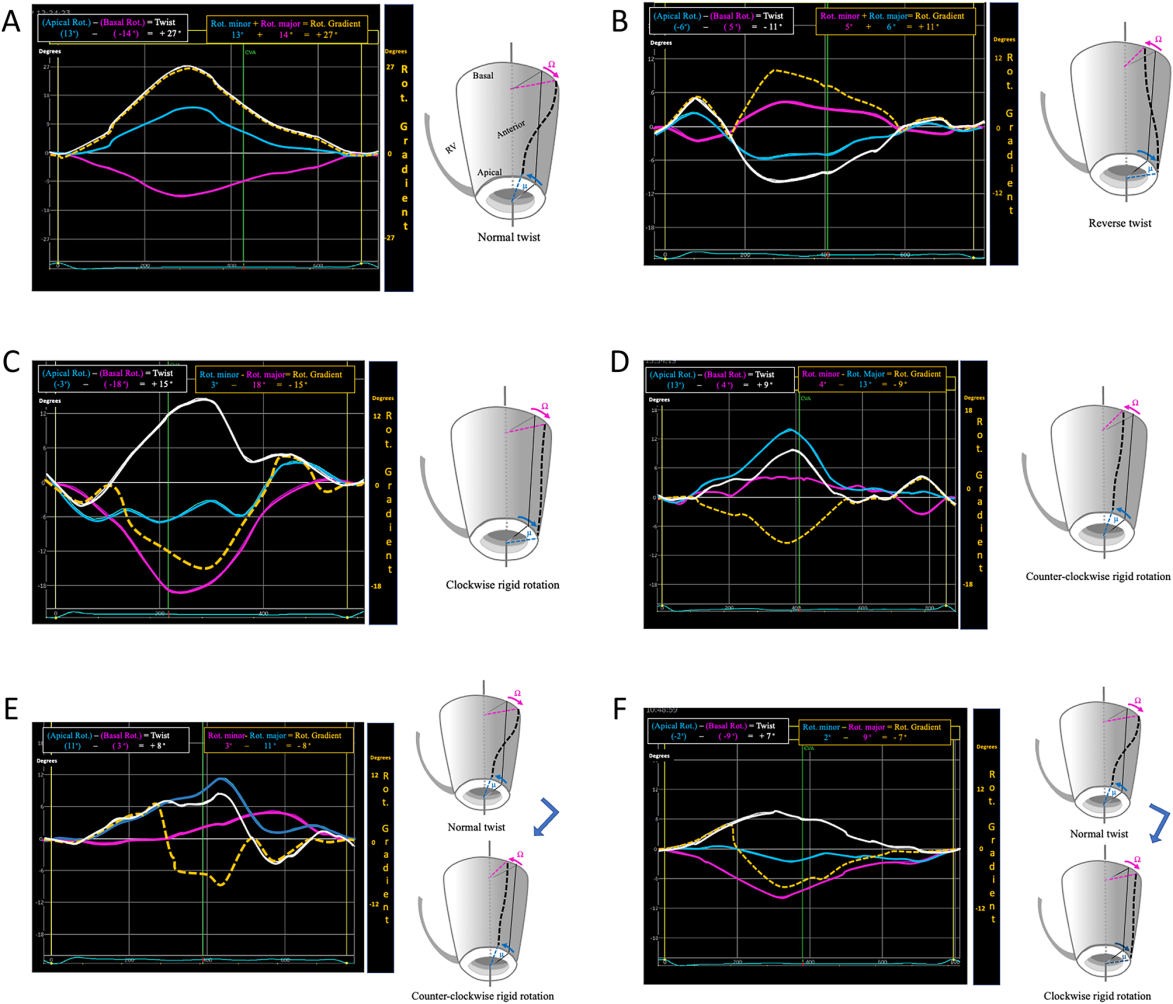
Examples of different rotation models obtained in our study population. Representation according to coding system. With the currently used coding system the curve of rotational gradient is represented as a continuous white line when visualized through an echocardiography device. With the coding system we propose, the curve of rotational gradient is represented as a broken yellow line (manual layout), and presents always as positive if there is twist and negative if there is rigid or semi-rigid rotation. Normal (A) and reverse (B) twist, which there is apical and basal rotation in opposite directions. Counter-clockwise (C) and clockwise (D) rigid rotation, which is produced when there is apical and basal rotation in the same direction (or absence of twist). Counter-clockwise (E) and clockwise (F) semi-rigid rotation, in which the apex and base initially rotate in opposite directions and eventually rotate in the same direction
